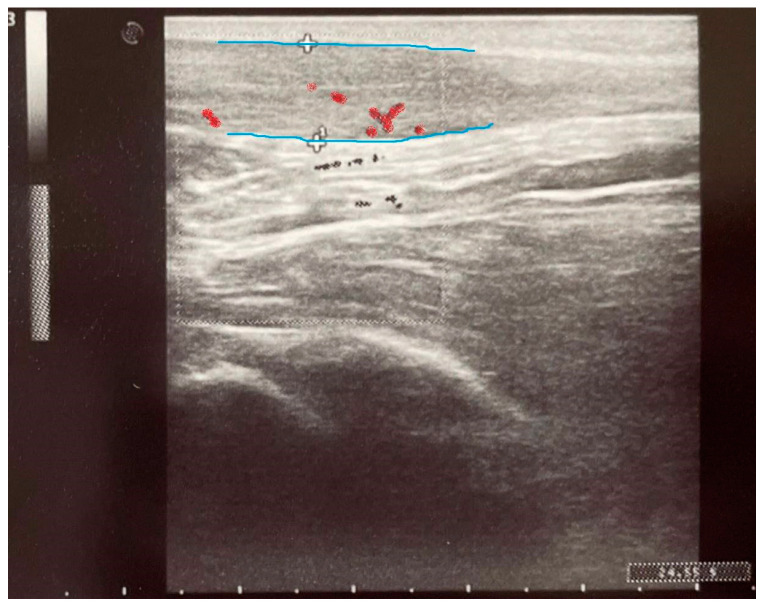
Longitudinal incidence ultrasound and color Doppler at 8 days showing fusiform thickening (blue lines) of the Achilles tendon with neovascularization (red)—diameter of 9.1 mm.
